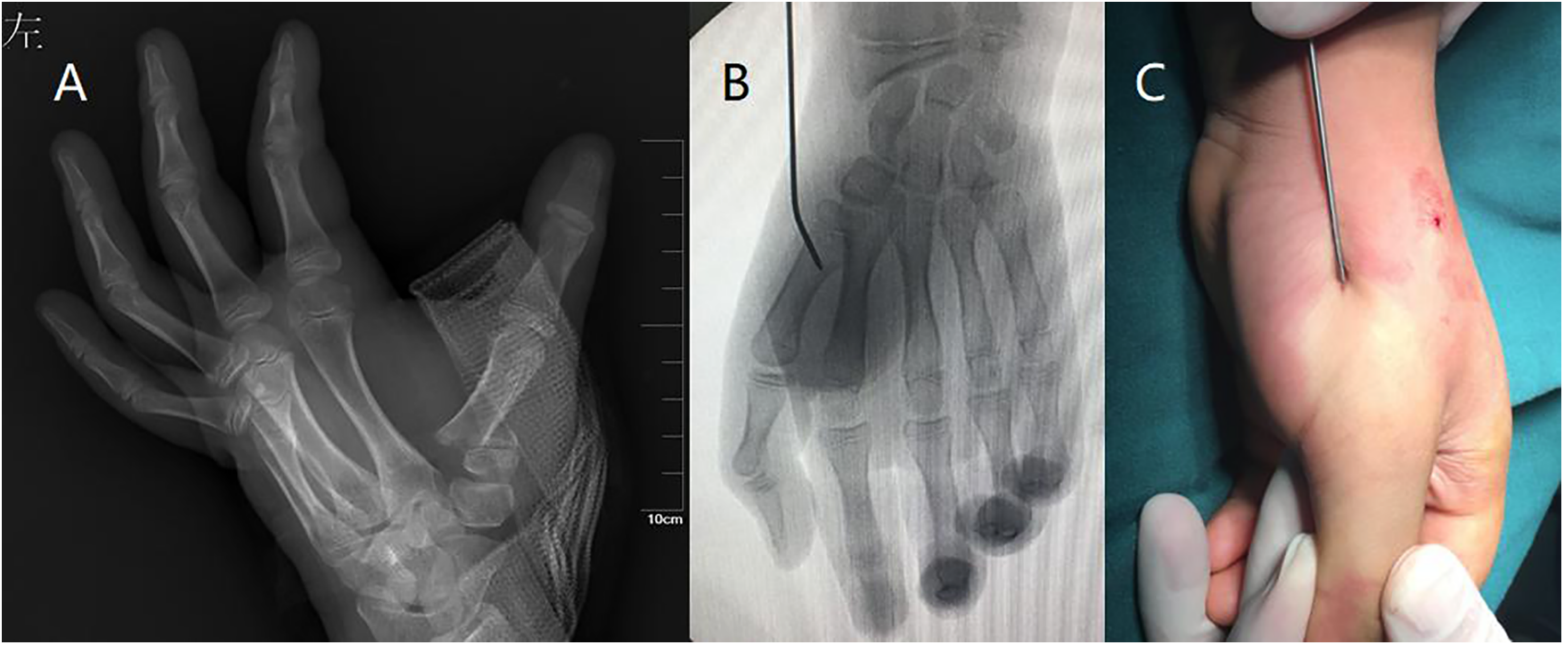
Typical thumb metacarpal base RW-C fracture of 10.5 years old boy. (A) AP x-ray of left thumb preoperative. (B) Closed reduction of the fracture. (C) Aspect of closed reduction.
